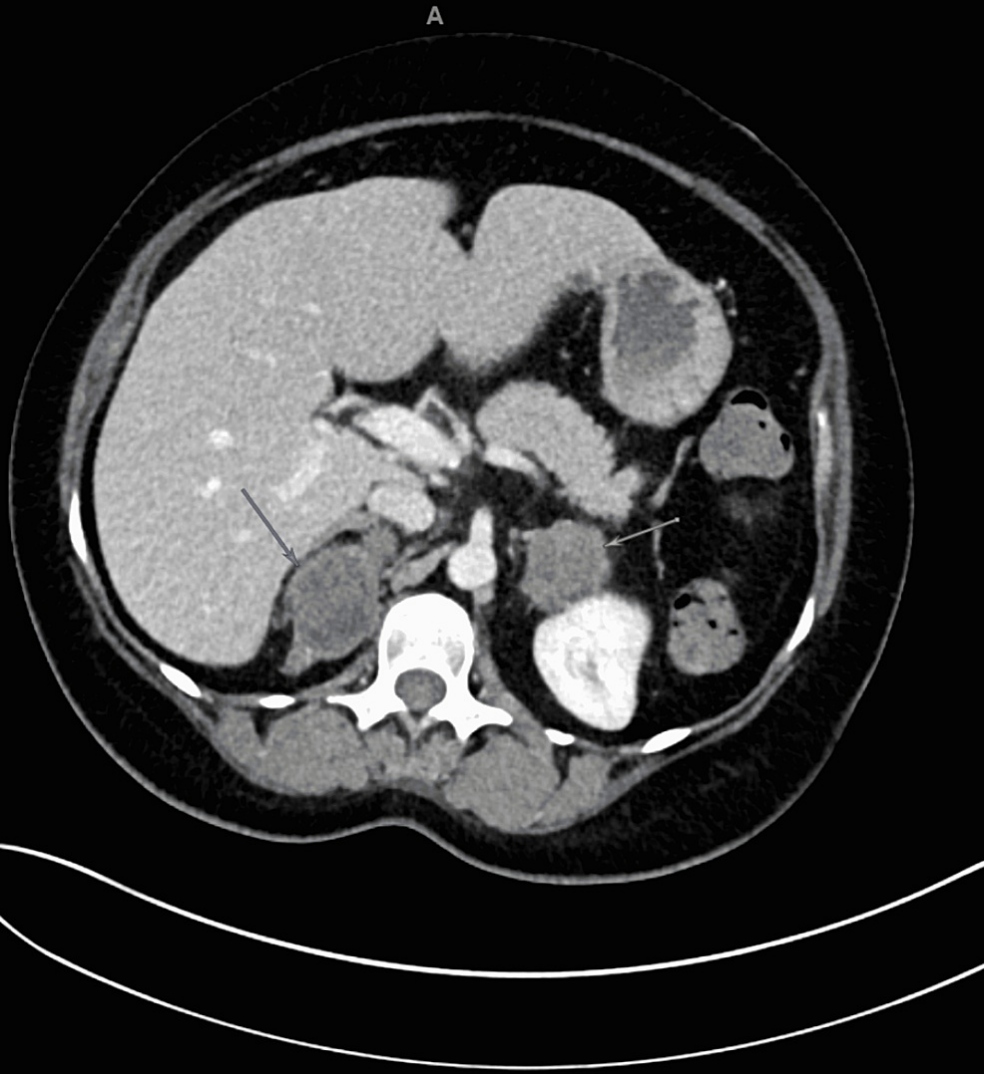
CT of the abdomen/pelvis with contrast showing low-attenuation masses present in both adrenal glands measuring 6.9 x 5.3 cm on the right (dark gray arrow) and 4.5 x 3.9 cm on the left (light gray arrow)
